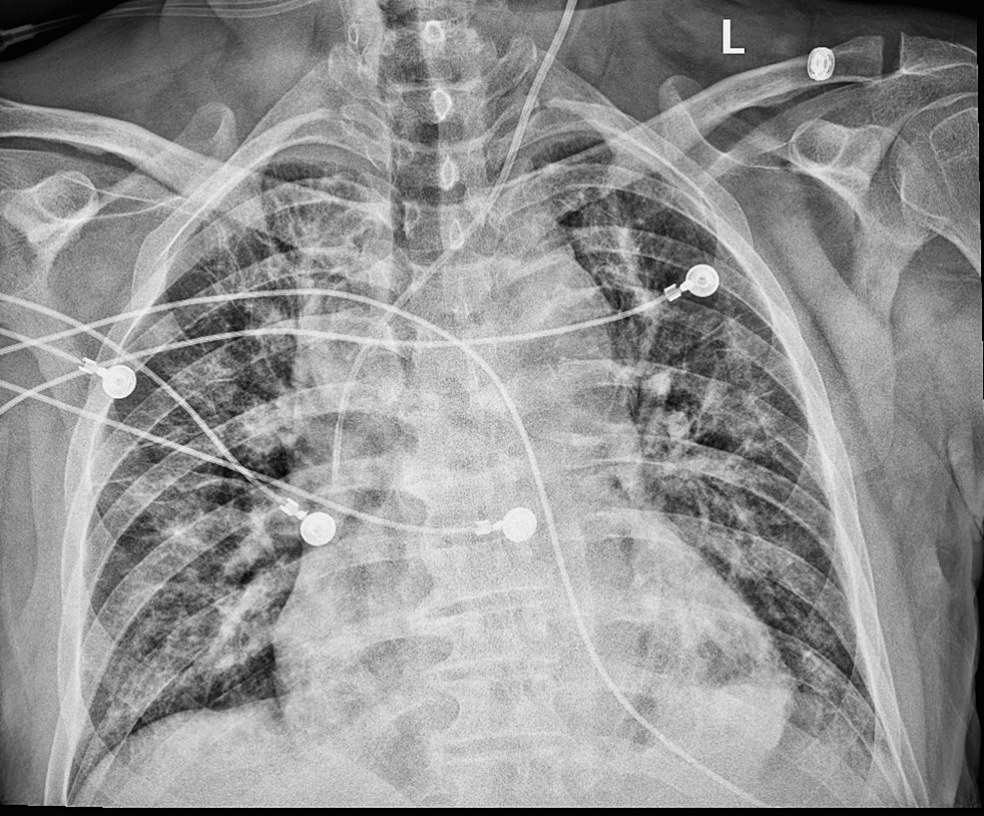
Chest x-ray performed at ED showing cardiomegaly and bilateral lung infiltrate ED: Emergency department
